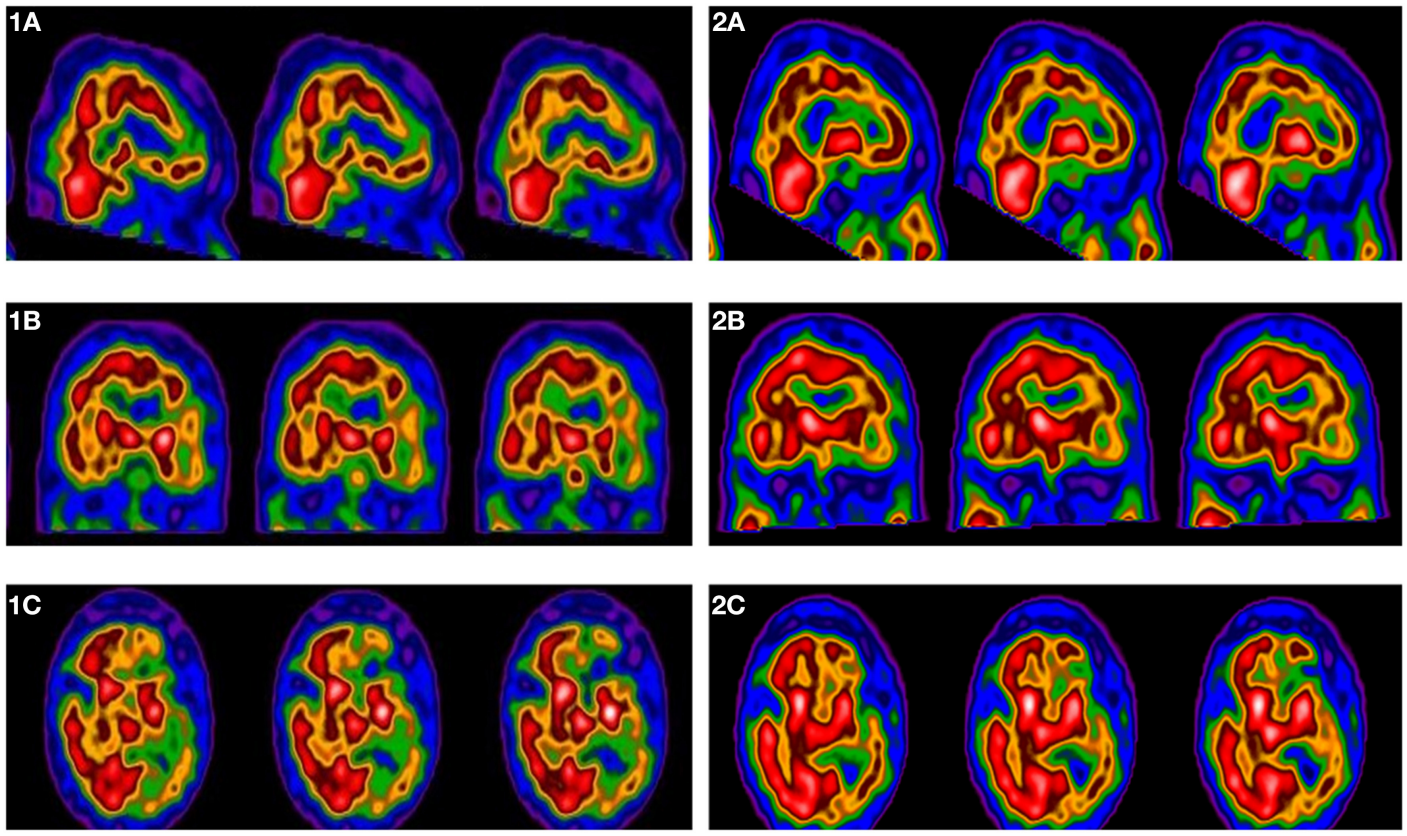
^99m^Tc-HMPAO brain perfusion SPECT scan at baseline in sagittal (1a), coronal (1b), and transaxial (1c) reconstruction; ^99m^Tc-HMPAO perfusion brain SPECT scan after fMV treatment in sagittal (2a), coronal (2b) and transaxial (2c) reconstruction. SPECT images show an increased ^99m^Tc-HMPAO distribution rate after fMV treatment in left frontal region (1a vs. 2a), in left parietal region (1b vs. 2b), in left occipital region (1c vs. 2c).
